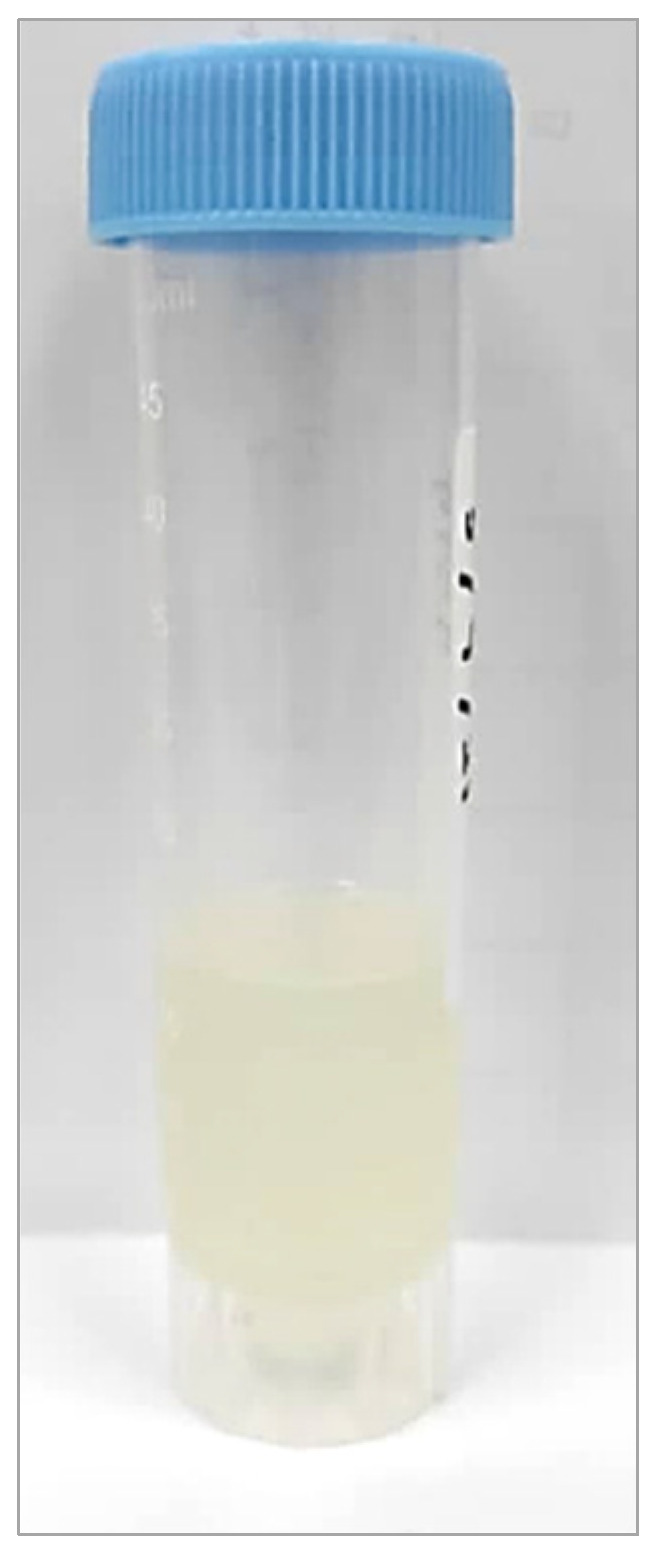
Nanoemulsion produced using ultrasonication method (NE-FLO).
